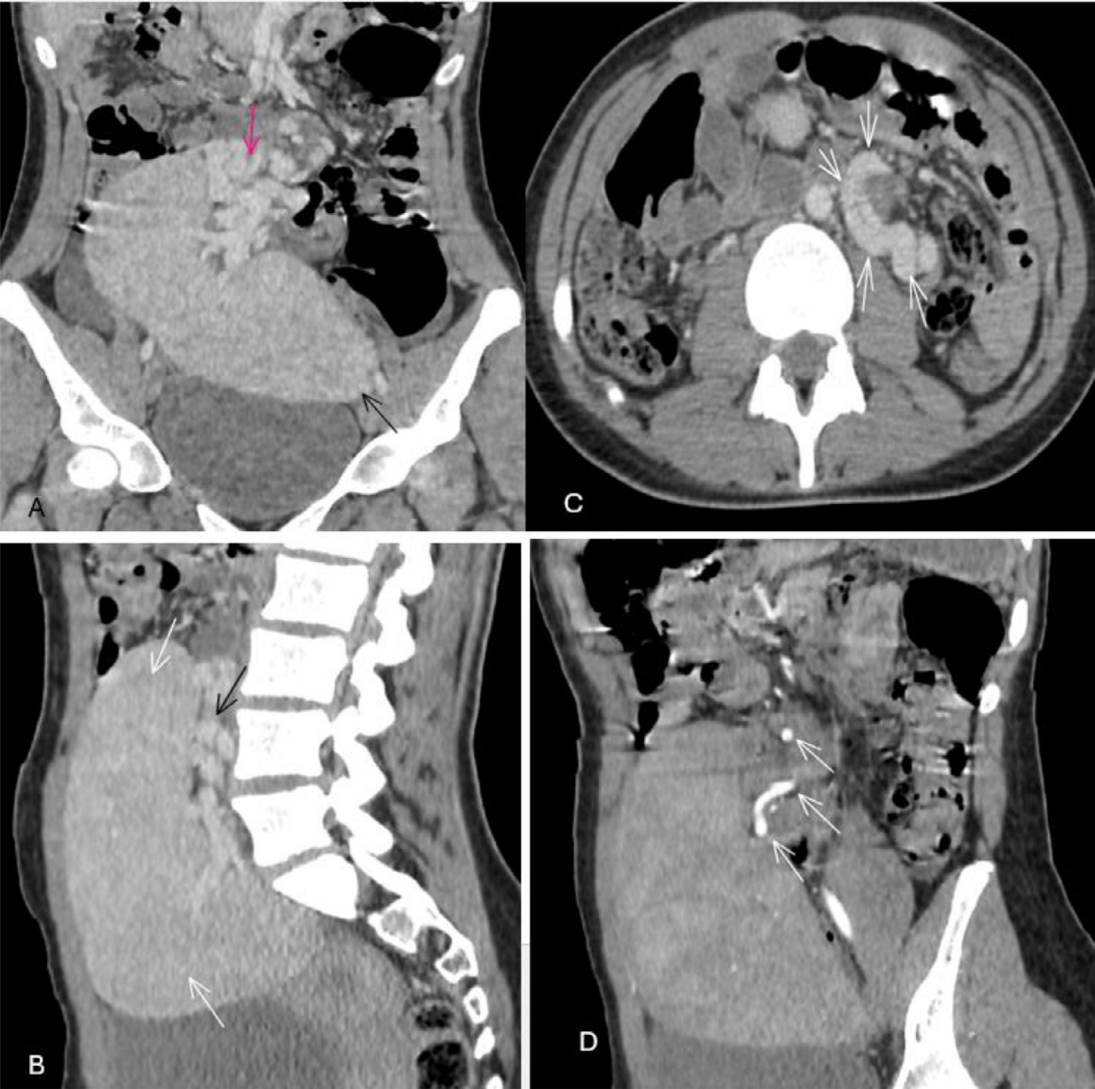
Post-IV abdominal CT images: venous phase (A-C) and arterial phase (D) showing an ectopic spleen located in the pelvic region. A long tortuous vascular pedicle containing the splenic vessels (C, white arrows: whirl sign) is seen extending from the epigastric region to the prolapsed spleen. There was a long splenic pedicle containing tortuous vessels with the splenic vein and artery. Sagittal contrast enhanced CT image showing a large ectopic spleen in the pelvis suspended by an elongated and tortuous vascular pedicle.
